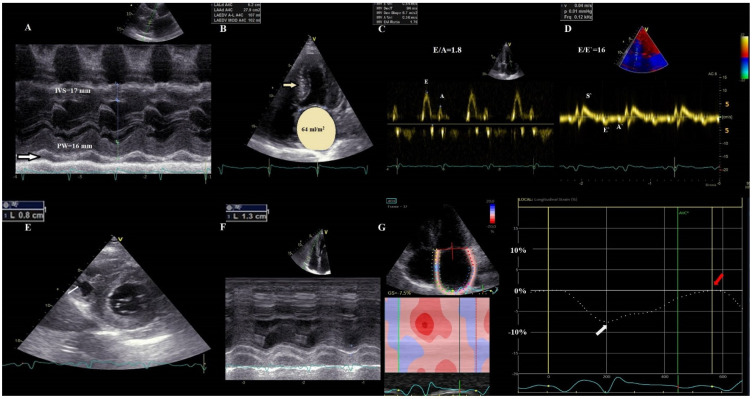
Transthoracic echocardiographic images in advanced ATTR-CM. (A) M−mode parasternal long-axis view of the LV displaying concentric severe LV hypertrophy (LV mass = 157 g/m^2^, RWT = 0.8), with mild pericardial effusion (white arrow); (B) apical 4-chamber view demonstrating a sparkling texture of the IVS and severely dilated LA (64 mL/m^2^); (C) Pseudo normal transmitral filling pattern (E/A = 1.8); (D) TDI tracing of the mitral annulus showing that all tissue velocities are <5 cm/s, with increased E/E’ ratio. (E) parasternal short axis view showing biventricular hypertrophy—RV free wall = 8 mm; (F) RV severe systolic dysfunction (TAPSE = 13 mm) (G) LA speckle-tracking showing significantly decreased pump function (white arrow) and even more severe reservoir function (red arrow), with the absence of conduit function. RWT, relative wall thickness, E = early filling velocity; A = late atrial filling velocity; S’, systolic TDI velocity; E’, early diastolic TDI velocity; A’, late diastolic TDI velocity; LA, left atrium; LV, left ventricular; RV, right ventricular; TAPSE, tricuspid annular plane systolic excursion; STE, speckle tracking echocardiography.
